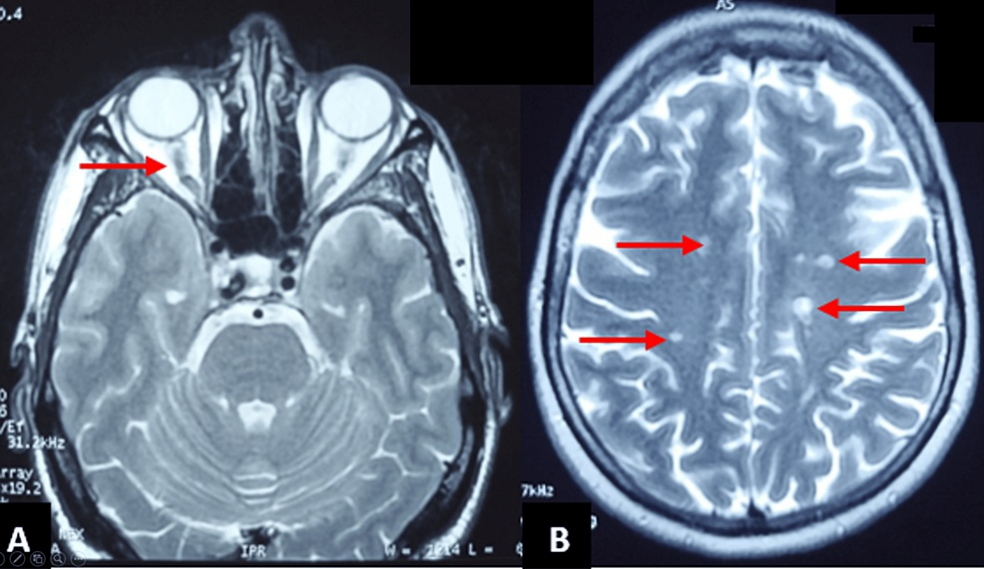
Brain and optic nerve abnormalities found in the first case admitted for acute vision loss associated with horizontal diplopia (A) Orbital MRI showing tortuosity in the optic nerves with bilateral optic neuritis (T2 sequence). (B) Subcortical white matter lesions in brain MRI (T2 sequence).
